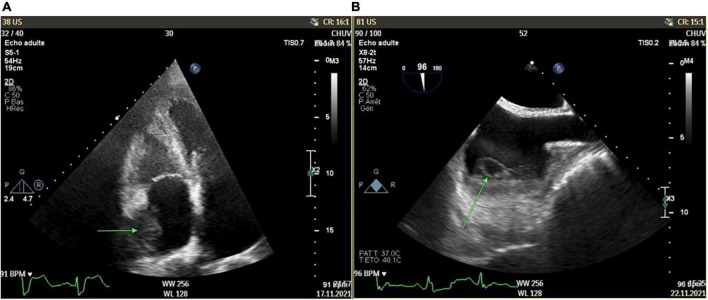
Right atrium mass (15 × 18 mm) with thickened lateral wall without associated tricuspid valve anomalies as shown in transthoracic echocardiography [green arrow, Panel (A)] and transesophageal echocardiography [green arrow, Panel (B)].
